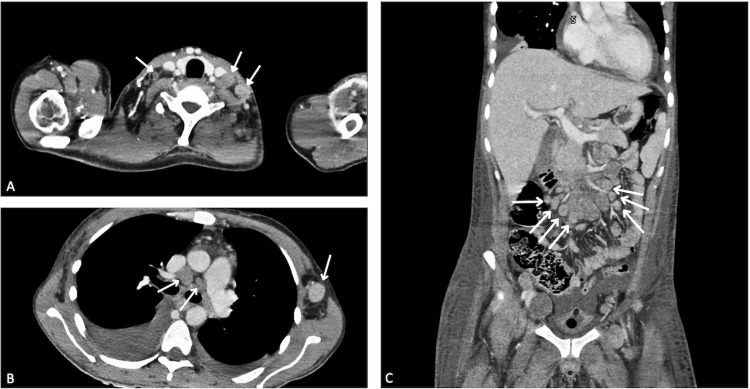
Contrast-enhanced computed tomography (CT) scan of the chest and abdomen Contrast-enhanced CT scan showing A and B: axial sections Arrows: cervical and mediastinal/axillary lymphadenopathy involvement. C: coronal section. Arrows: retroperitoneal lymphadenopathy
